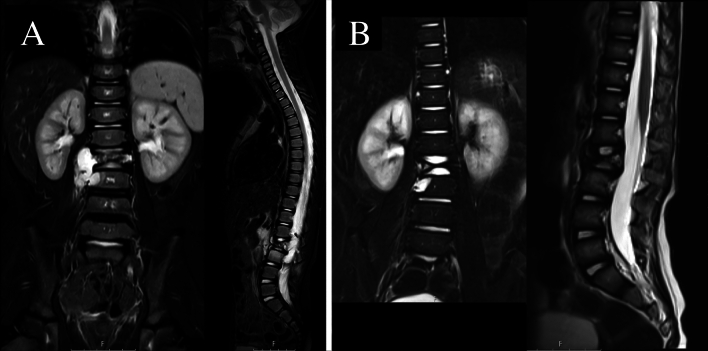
a MRI showing a 12-year-old male patient affected by a Ewing Sarcoma of L2-L3 vertebrae with intracanal compromission. b the same patient after neoadjuvant chemotherapy; the extraosseous component was no longer present
